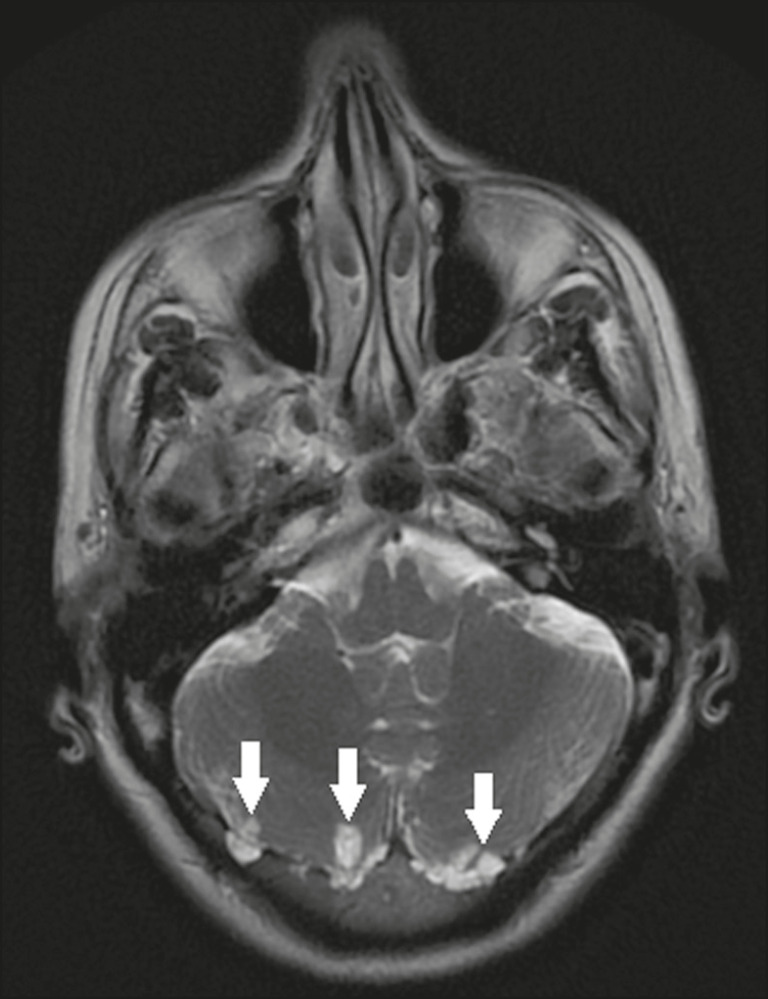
Arachnoid granulations. Axial T2-weighted MRI showing rounded, cyst-like lesions, located extra-axially, with intensity similar to that of the CSF, in close proximity to the cerebellar hemispheres (arrows).
